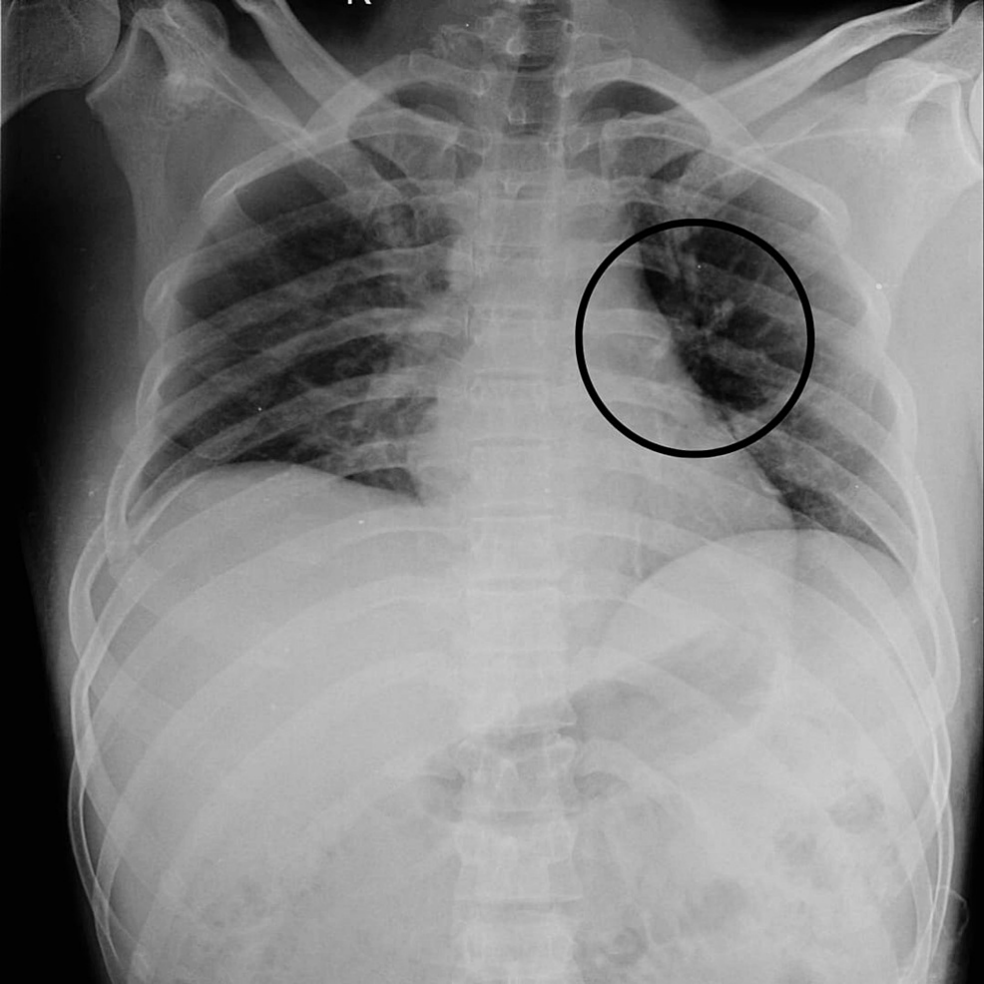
X-ray of chest showing fourth, fifth, and sixth rib fracture The circle shows a fracture of the anterolateral aspect of the right fourth and fifth ribs and the posterolateral aspect of the sixth rib.
